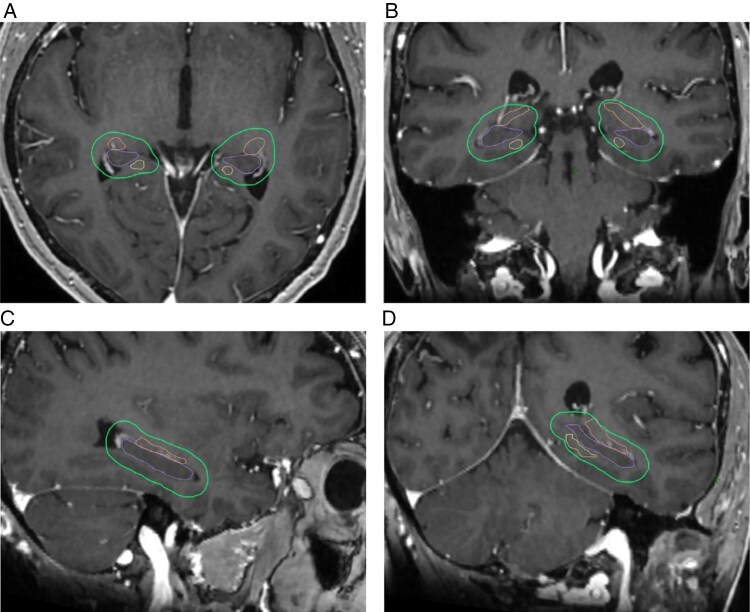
MRI of a brain with the anatomical borders of the hippocampus (purple), fornix (orange), and parahippocampal cingulum (PHC, yellow) with a 5 mm margin (green) in an axial (2A), coronal (2B), sagittal (2C), and oblique (2D) view.
